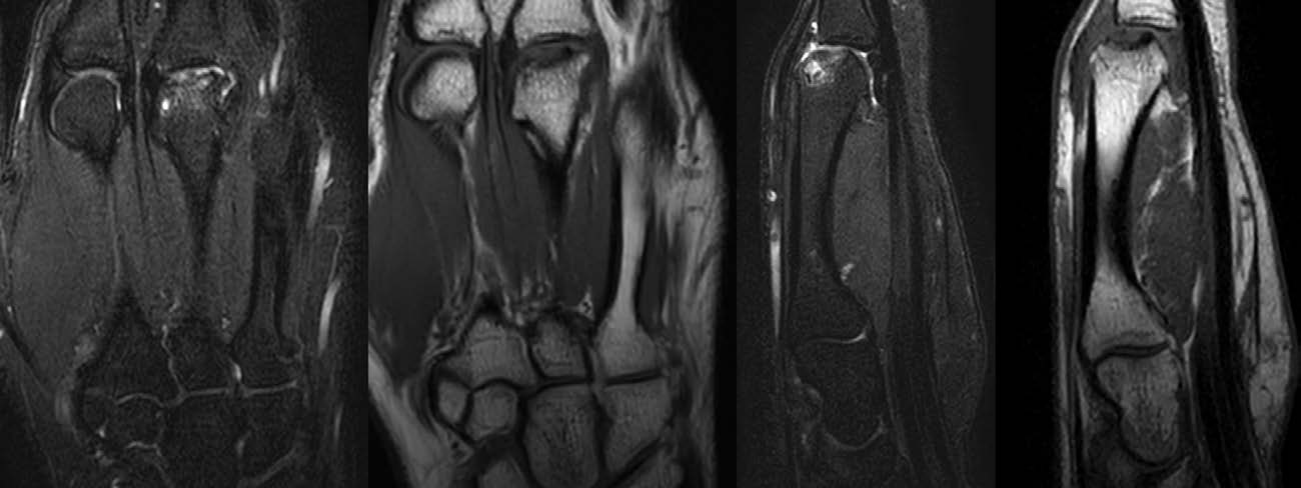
Magnetic resonance image scans of the right hand demonstrating articular cartilage wear, subchondral edema and signal change consistent with avascular necrosis of the 3rd metacarpal head.
